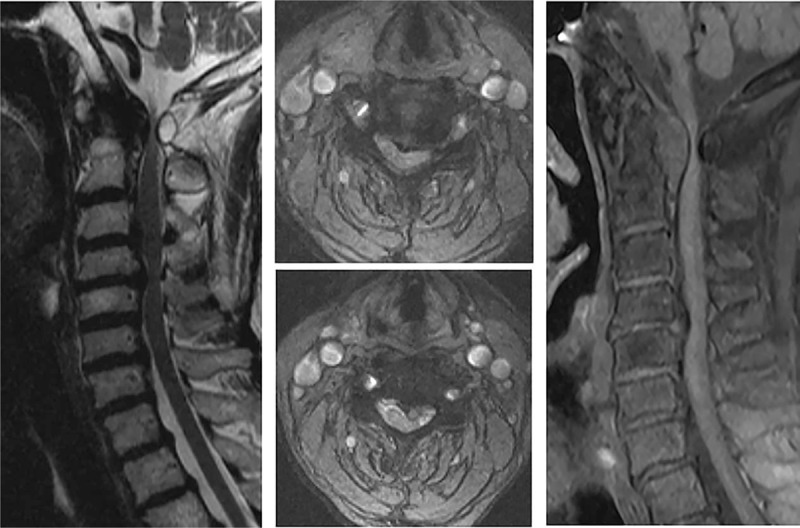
Magnetic resonance imaging and enhanced magnetic resonance imaging revealed hypertrophy of posterior longitudinal ligament at C1-2 and consecutive spinal canal stenosis from C3 to C6 levels. Spinal cord was compressed at both upper and lower level.
